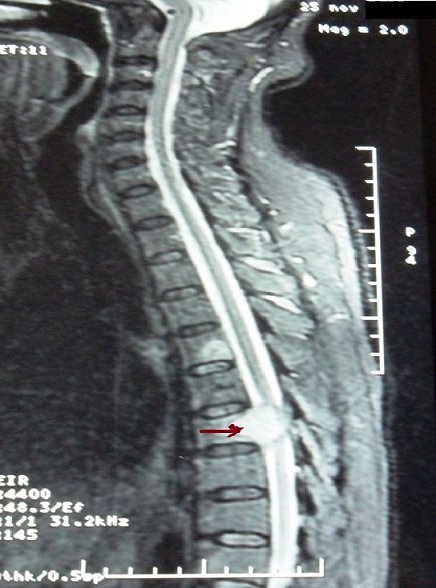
Spinal MRI, sagittal view: spinal cord compression in relation to the seventh dorsal vertebra
